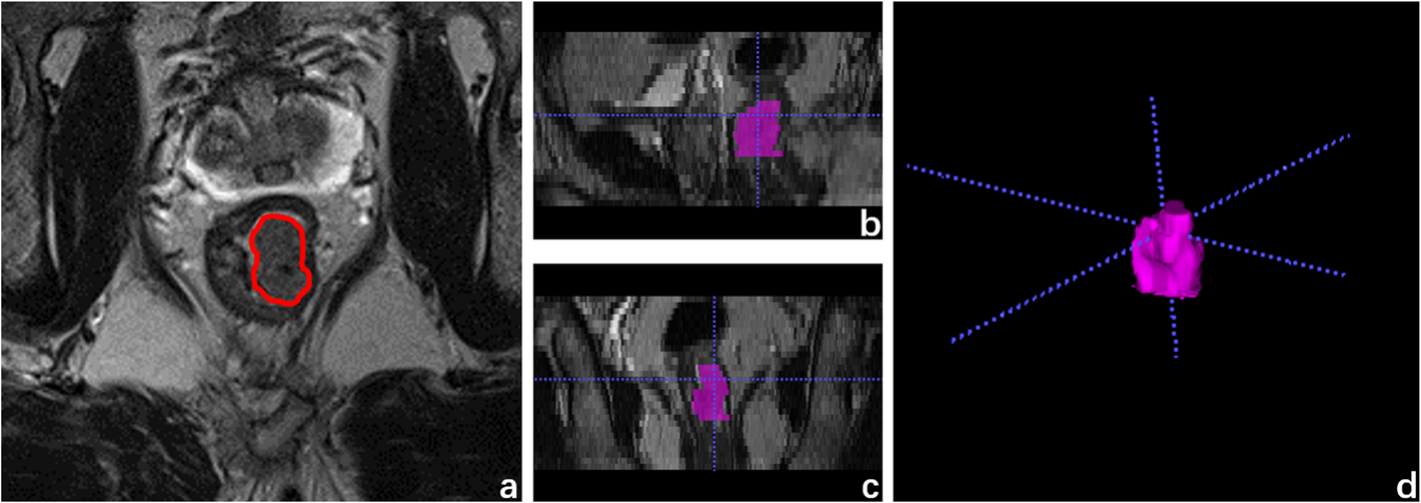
Example image for rectal cancer contouring. a The outline of ROI on one slice of axial T2-weighted MR image. b Sagittal reconstruction. c Coronal reconstruction. d Volume rendering
